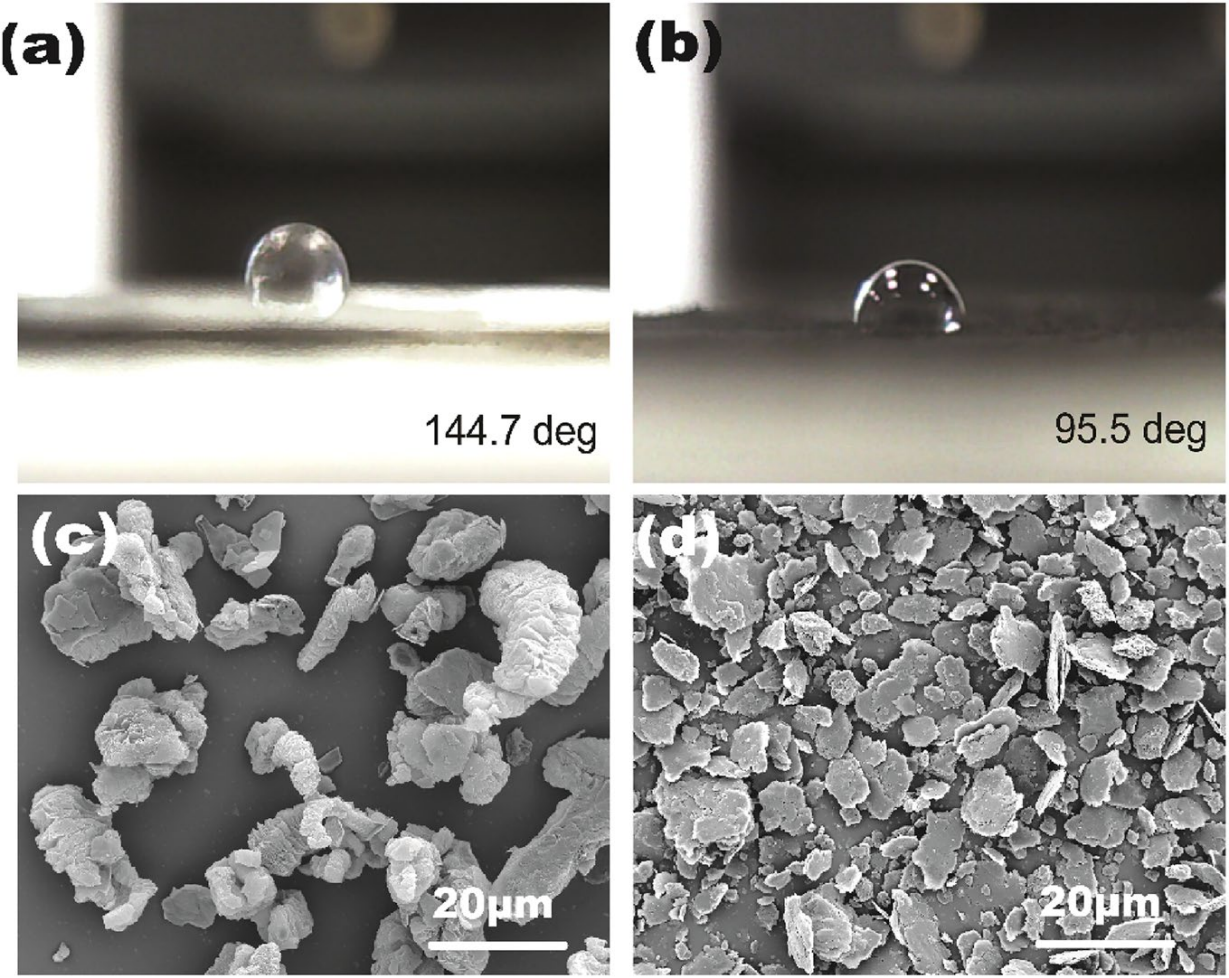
Side-view optical images of water-drop on (a) original graphene film and (b) ultrasonic treated graphene film. SEM images of the original graphene (c), after ultrasonic treated graphene (d).
